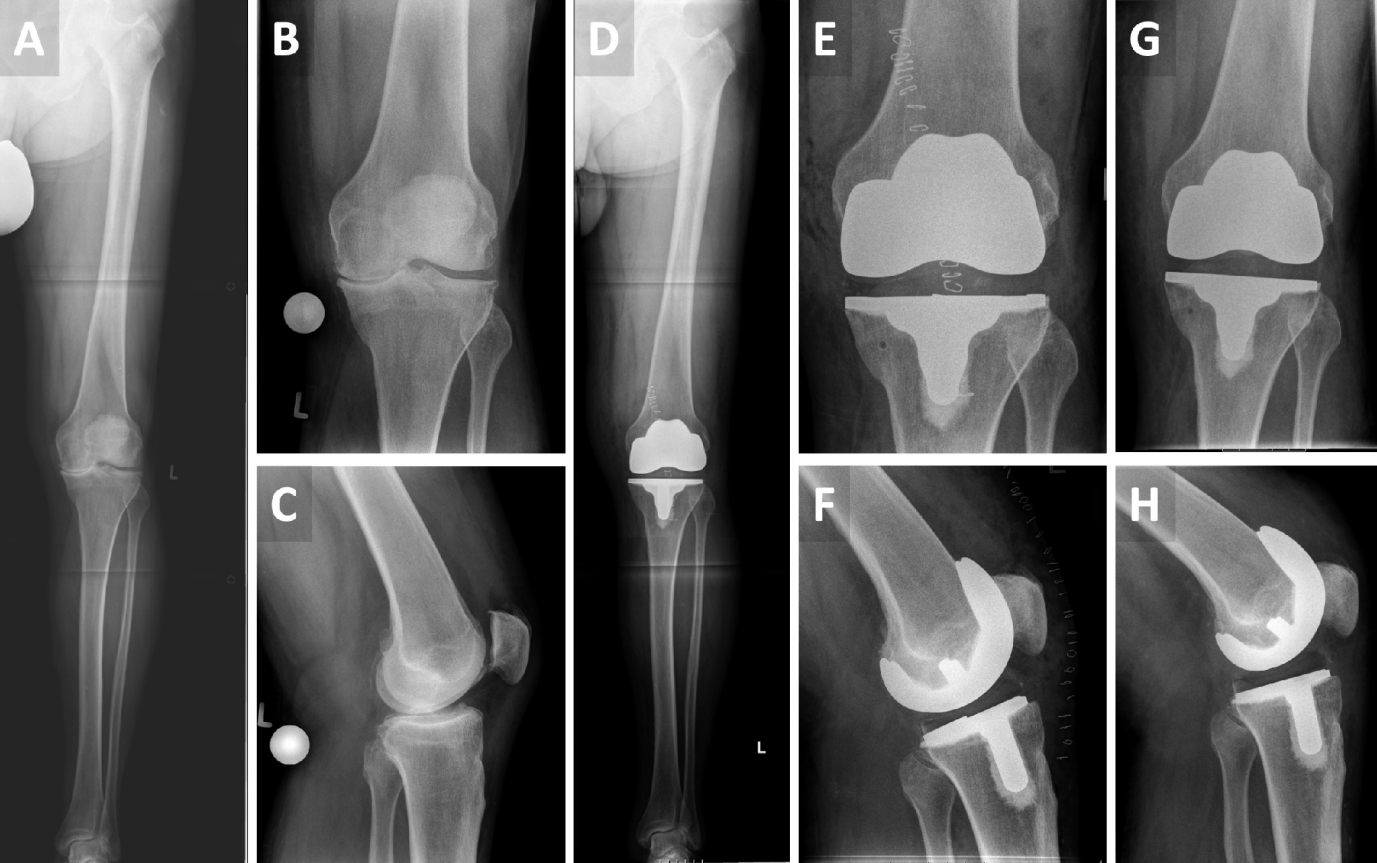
Exemplary radiographic imaging A, B, C: preoperative radiographs D, E, F: immediate postoperative radiographs G, H: radiographs at last follow-up
